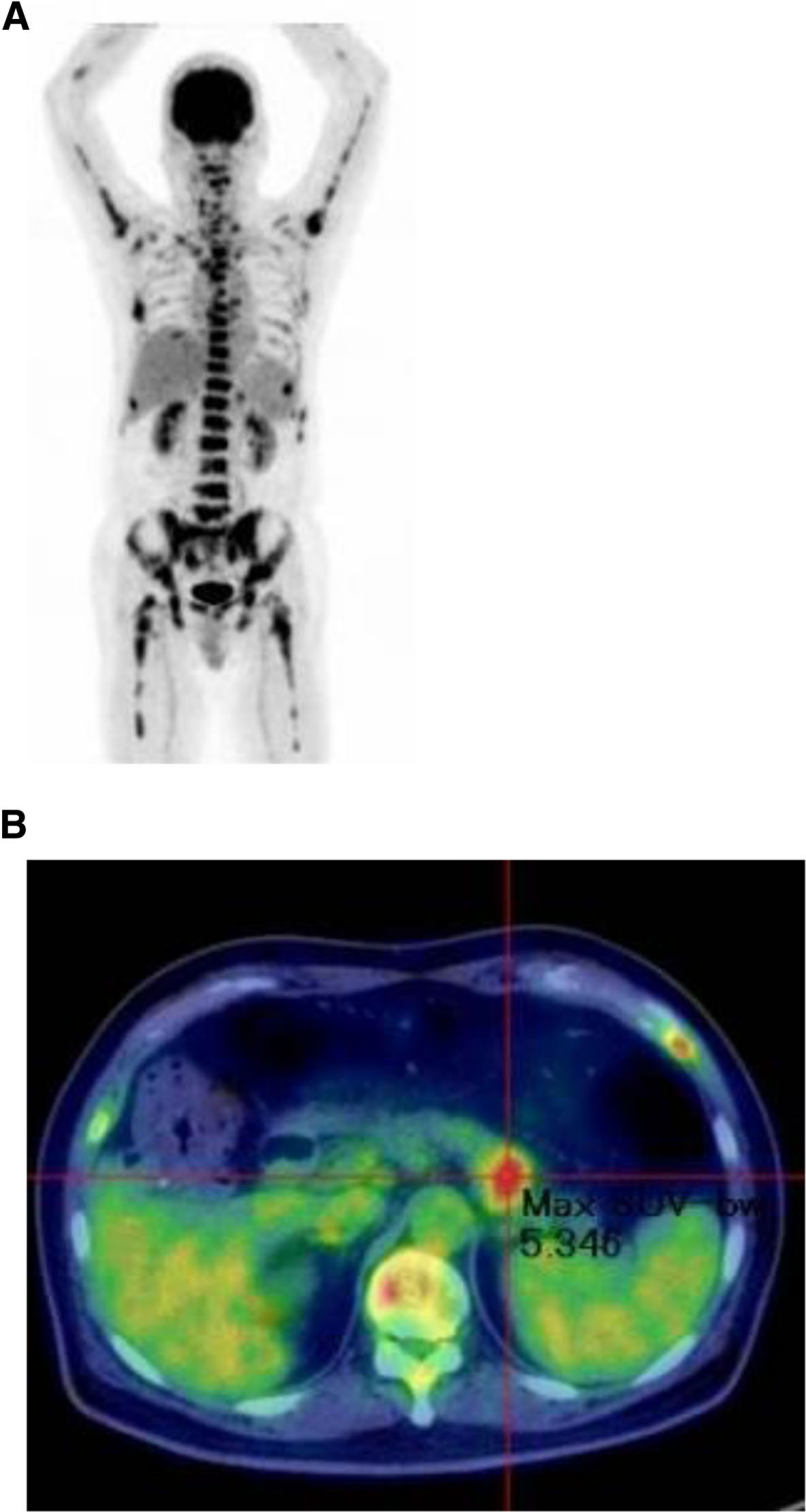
PET-CT studies. a: Diffuse skeletal uptake; b: Abnormal uptake in the tail of the pancreas
